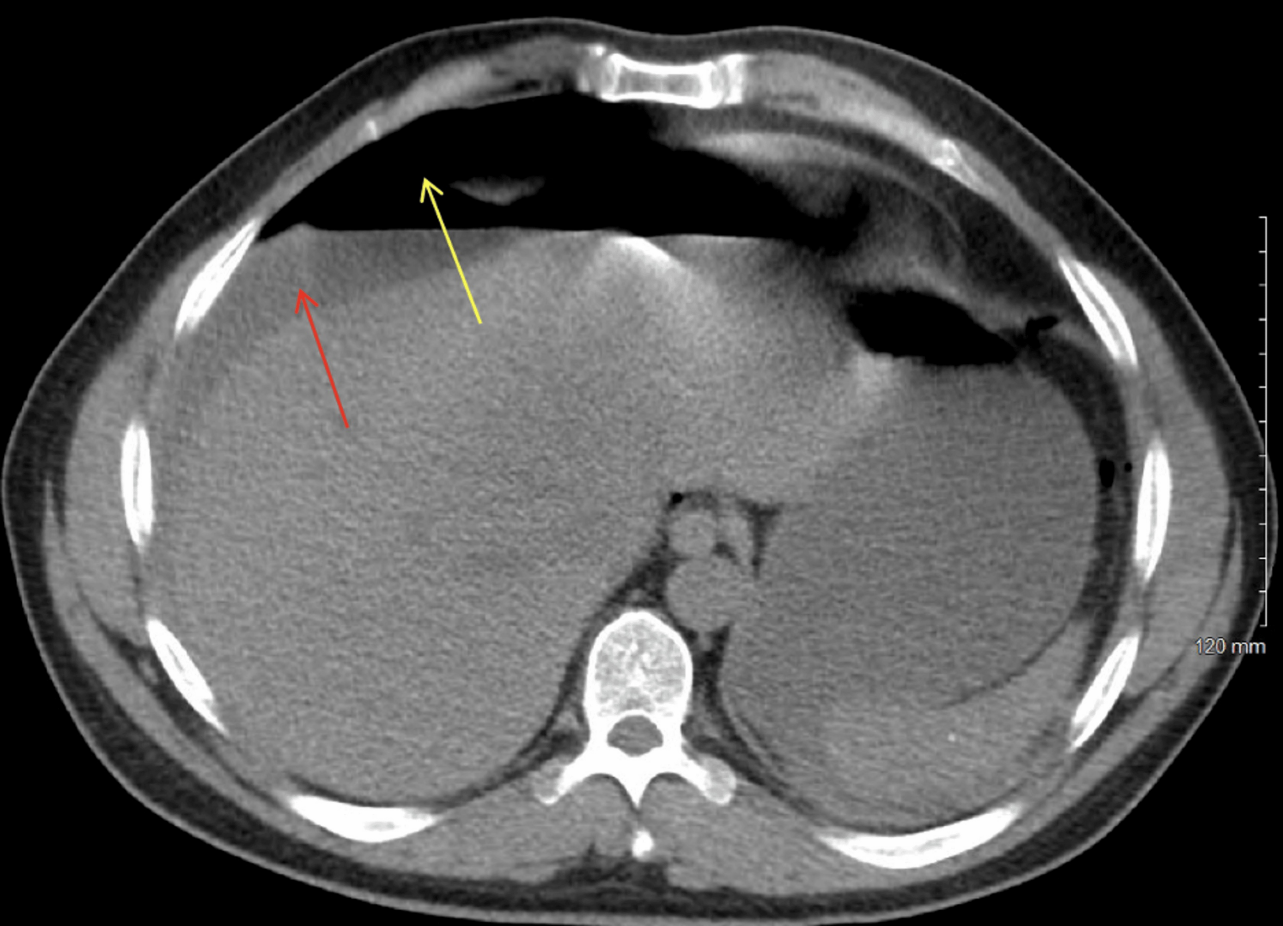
CT scan of the abdomen and pelvis without contrast (axial view) demonstrating a substantial amount of free intraperitoneal fluid (indicated by the red arrow) and extensive pneumoperitoneum (indicated by the yellow arrow). These findings are highly concerning for a perforated viscus.
